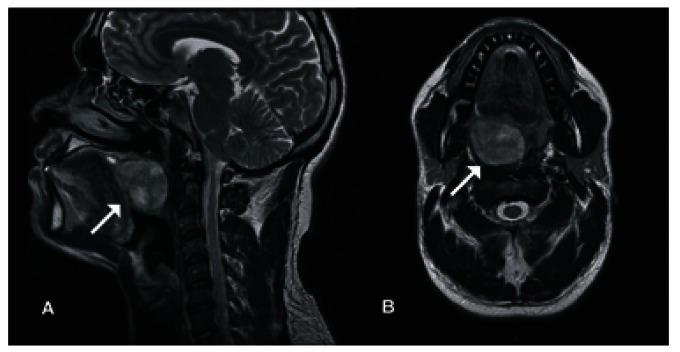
A) Sagittal MRI showing lesion in the soft palate and there is total obstruction of the naso-oropharynx. B) Axial MRI with lesion found to the right of the midline dependent on the soft palate.
